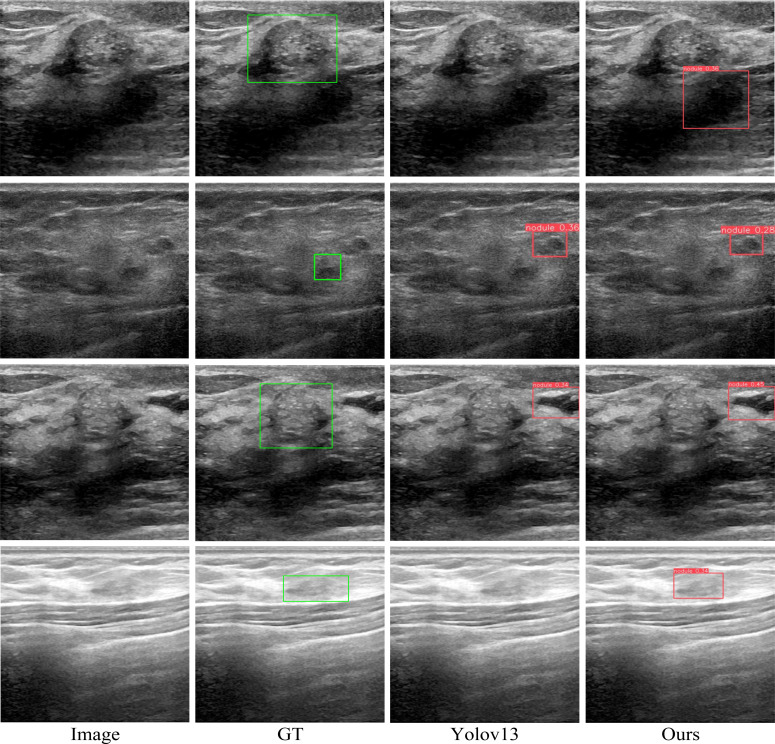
Representative failure cases on challenging breast ultrasound images.
